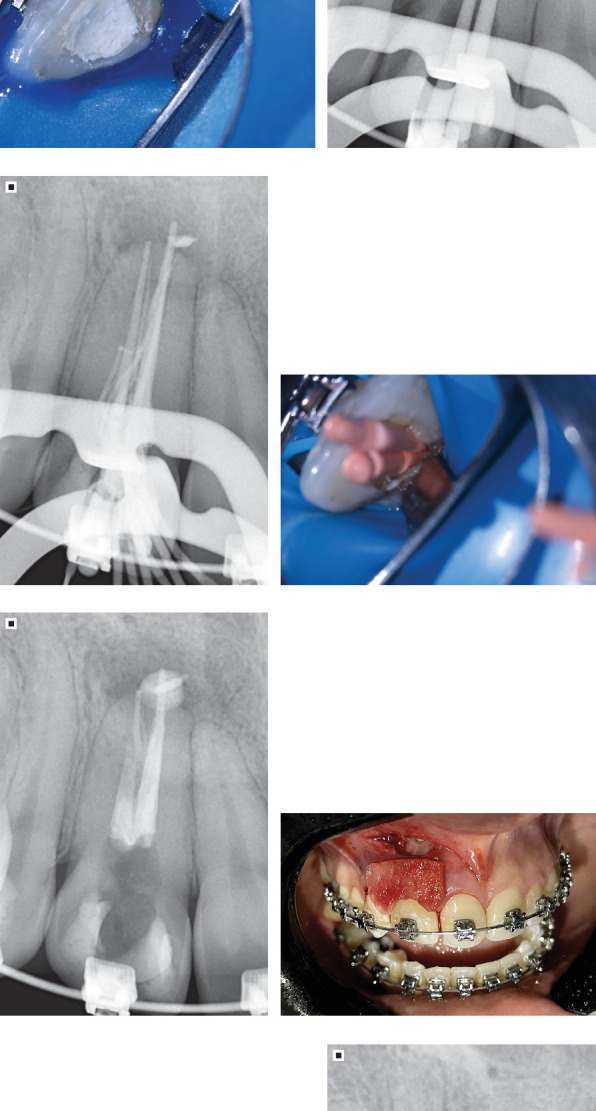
(a) Occlusal view, initial aspect after coronary access. (b) Radiographic aspect of Tooth 12 during removal of obturation material and canal decontamination process. (c) Occlusal view, completion of the first clinical session in which gutta-percha removal and root canal instrumentation were performed, showing restoration with provisional zinc oxide and eugenol cement (IRM, Dentsply Sirona) during the 7-day intervals between sessions in which intracanal medication was applied. (d) Periapical radiograph of the gutta-percha cone fitting. (e) Periapical radiograph prior to filling the root canal. (f) Occlusal view during the final clinical session where instrumentation was completed and root canal filling performed. (g) Periapical radiograph showing the final aspect after endodontic retreatment. (h) View of the dermal acellular matrix before stabilization with sutures. (i) Final presentation after stabilization of the dermal acellular matrix using simple and simple and suspension sutures. (j) Periapical radiograph showing the final aspect after periradicular surgery due to the radicular cyst, diagnosis confirmed postoperatively by oral and maxillofacial pathology laboratory. (k) Occlusal view after definitive restoration of coronary access.
